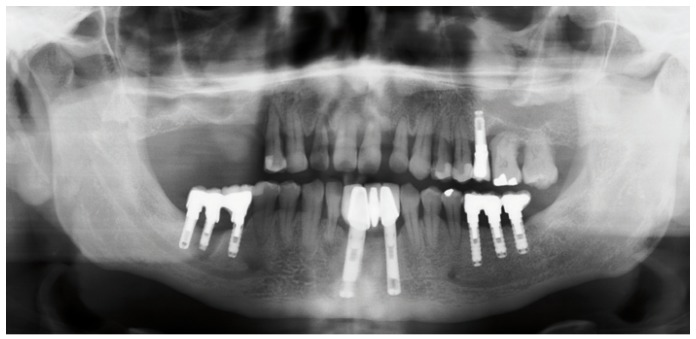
Case 2. 6. Preoperative panoramic X-ray view.
